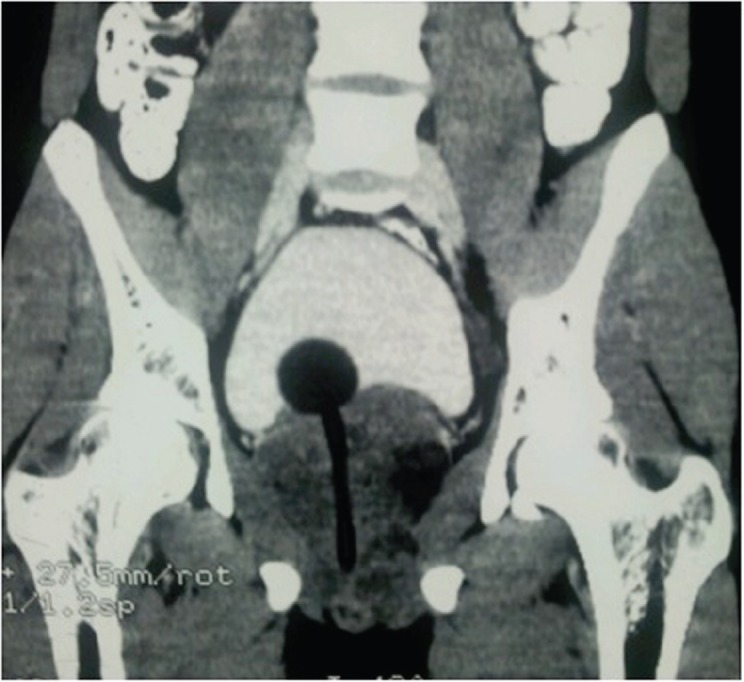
CT scan of the pelvis showing heterogeneously enhancing mass replacing the prostate infiltrating the bladder base and Foley’s bulb and catheter *in situ*.
